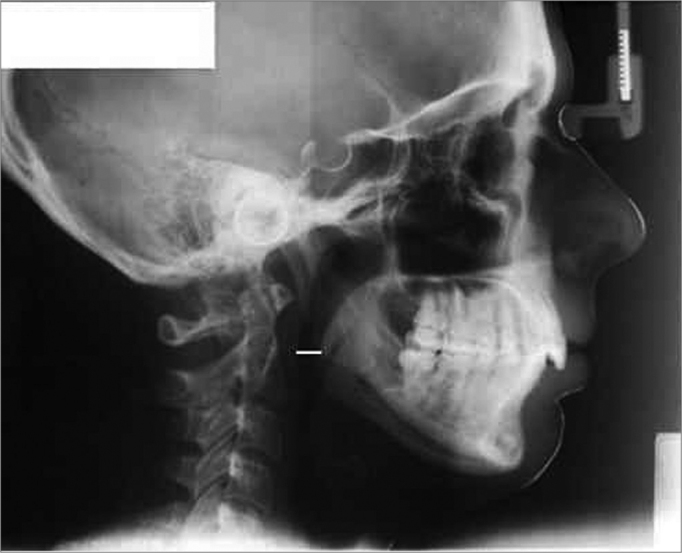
PAS (lower airway) Cephalometric value: pharynx width at the point where, radiographically, the posterior tongue border crosses the inferior mandible border, all the way to the closest point to the posterior pharyngeal wall.
